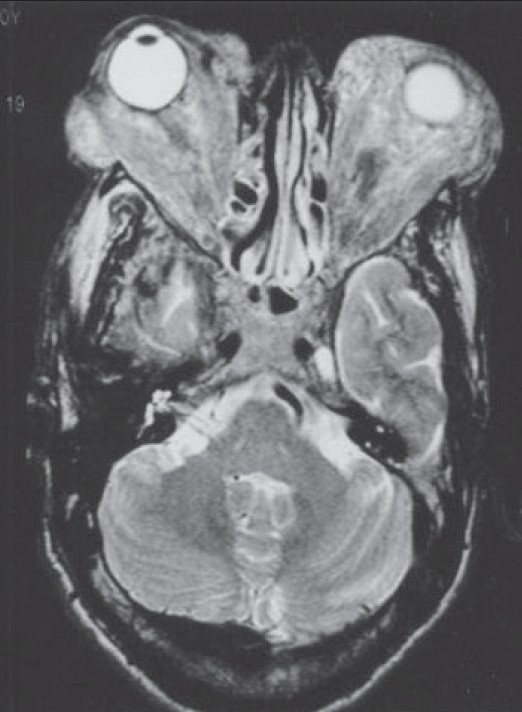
The orbital MRI reveals bilateral advanced proptosis with diffuse infiltration of orbital fat, obliteration of optic nerves, extraocular muscles with fi xation of intraorbital structures and enlarged lacrimal glands. Extraocular muscles show diffuse enlargement (both tendons as well muscle bundles enlarged in a tubular confi guration), with involvement of perineural optic sheath
